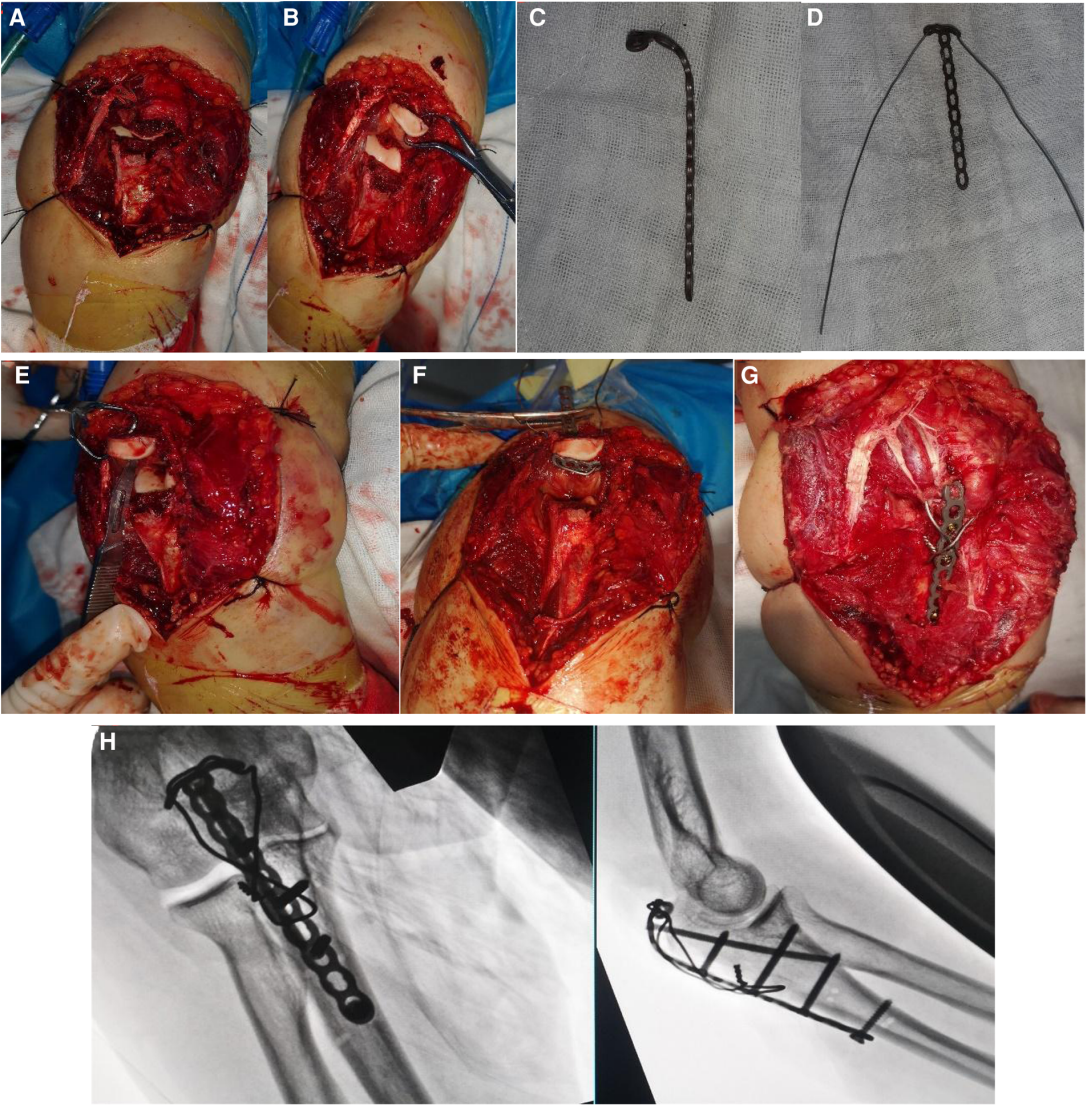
(A,B) Approach to the fracture; (C,D) bending the T-shaped plate and attaching the steel wire; (E) small incision in the triceps tendon; (F) passing the plate from the inside to the outside, thus making the T-shaped end to lean on the bare bone area of the olecranon; (G) pulling the triceps brachii tendon and fixing the fracture; (H) final operative x-ray.
